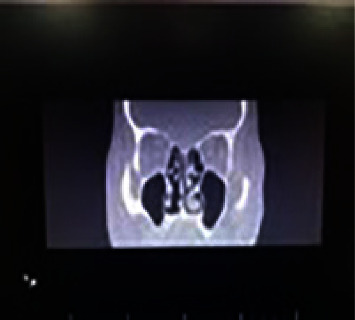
Normal paranasal sinuses CT scan.
